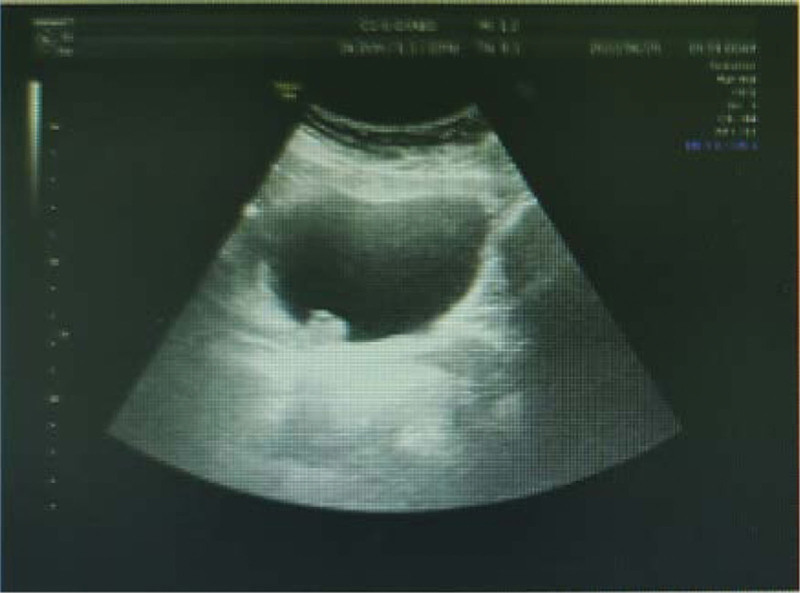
The urinary tract ultrasound. A strong echo of 2.28 × 1.99 cm in size was observed at the right ureteral entrance. The strong echo had clear boundary, irregular shape, no obvious blood flow signal, and no obvious movement even when the position was changed.
